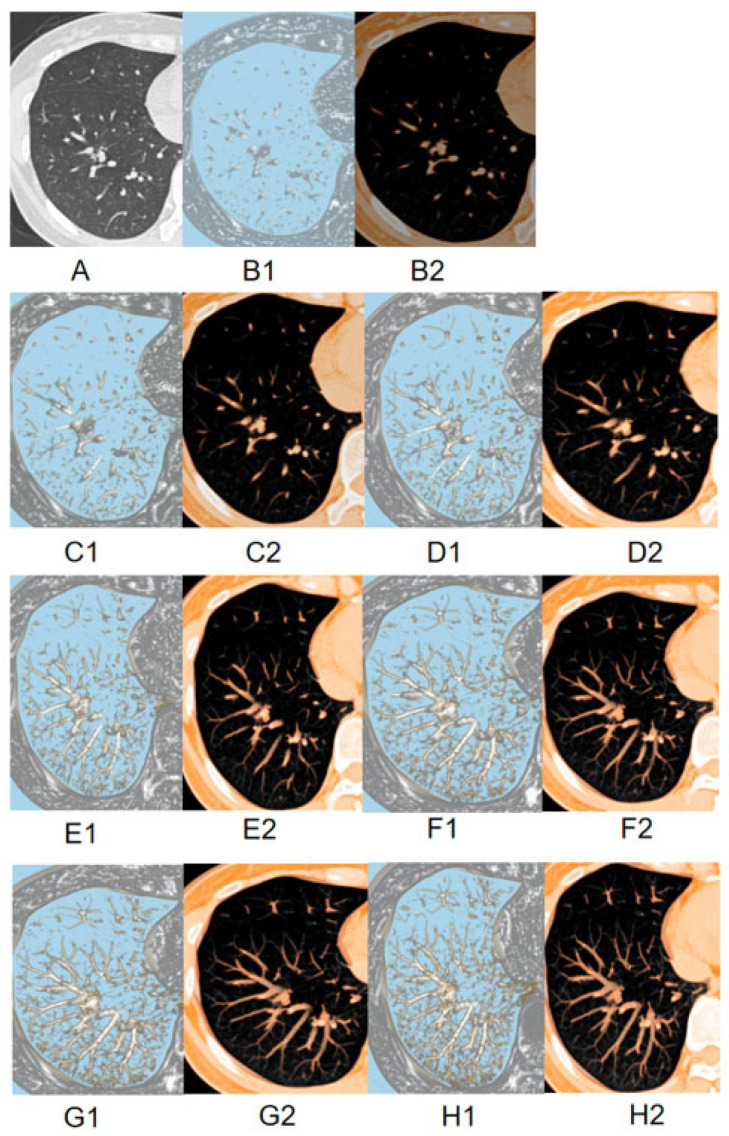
The image displays two-dimensional TSCT images (A); 2 mm MPVR model (B); 4 mm MPVR model (C); 6 mm MPVR model (D); 8 mm MPVR model (E); 10 mm MPVR model (F); 12 mm MPVR model (G); 14 mm MPVR model (H) from the CT dataset of a 57-year-old patient with a part-solid pulmonary nodule. For each thickness, the left panel (B1–H1) shows the nodule detection plane, and the right panel (B2–H2) shows the orthogonal plane for assessing the part-solid components.
